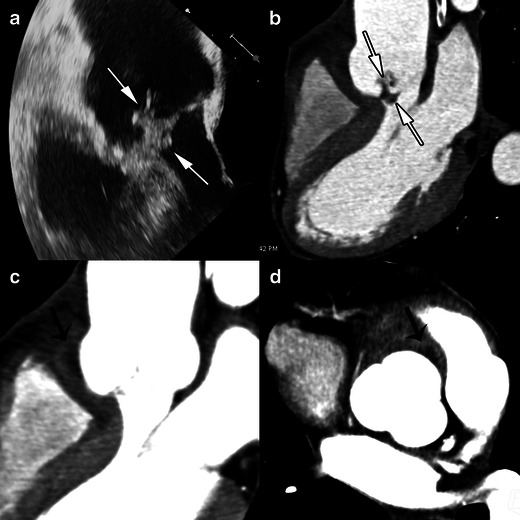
Results of echocardiography and MSCT studies in a case of aortic valve IE. The TEE study, 120-degree long-axis view (a) and MSCT acquisition with MPR reconstructions in the LVOT view (b), show a huge vegetation on the aortic valve (*white arrows*). MSCT acquisitions with MPR in the LVOT view (c) on the level of aortic annulus (d) are displayed in a narrowed imaging window, suitable for analysing para-valvular tissue, and show an abscess located in the anterior part of the aortic root (*black arrows*)
